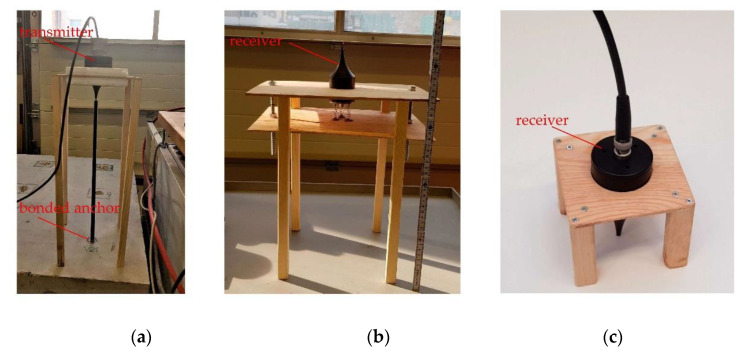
Test setups for different ultrasonic measurement configurations: (a) test setup for all types of transmission, top side; (b) test setup for direct transmission, bottom side; (c) test setup for indirect transmission, top side.
